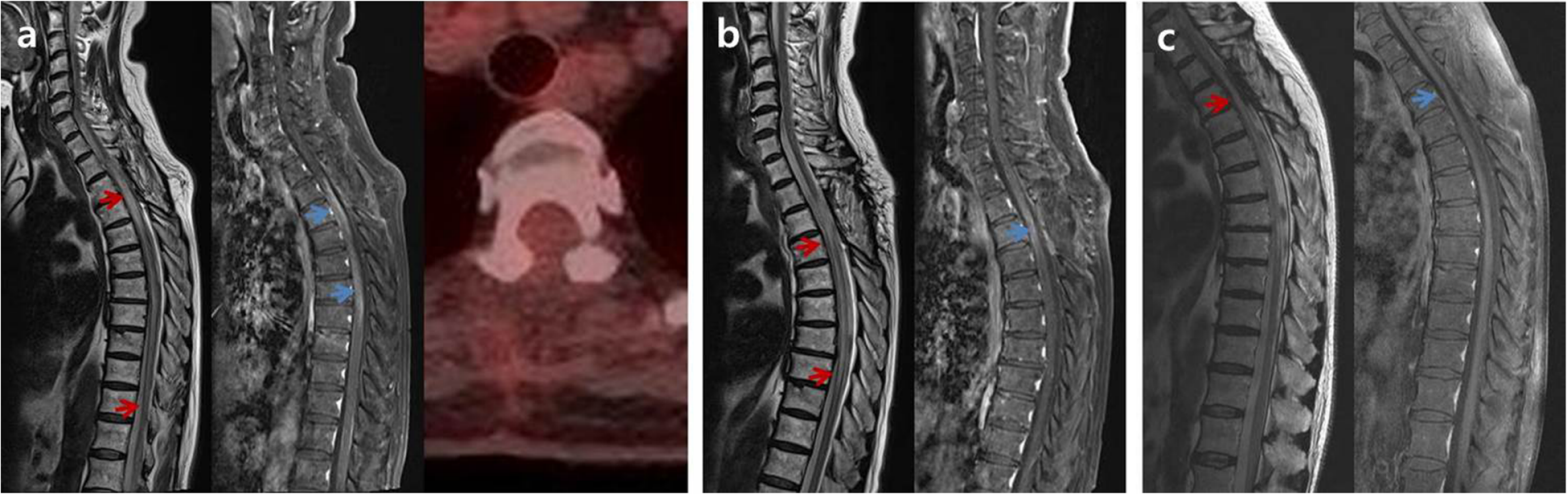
On the initial radiological findings after development of paraplegia at 18-month follow-up (a), magnetic resonance imaging (MRI) showed swelling, extensive edema (red arrows), and contrast enhancement (blue arrows) of whole thoracic cord including previous tumor surgical lesion on T2-4. However, there is no evidence of recurrence or new lesion on whole-body ^18^F-fluorodeoxyglucose positron emission tomography/computed tomography. On the 2-week follow-up MRI, there was significant reduction of edema and contrast enhancement in thoracic cord (red and blue arrows) (b), which was almost disappeared on the 5-week follow-up MRI (c)
